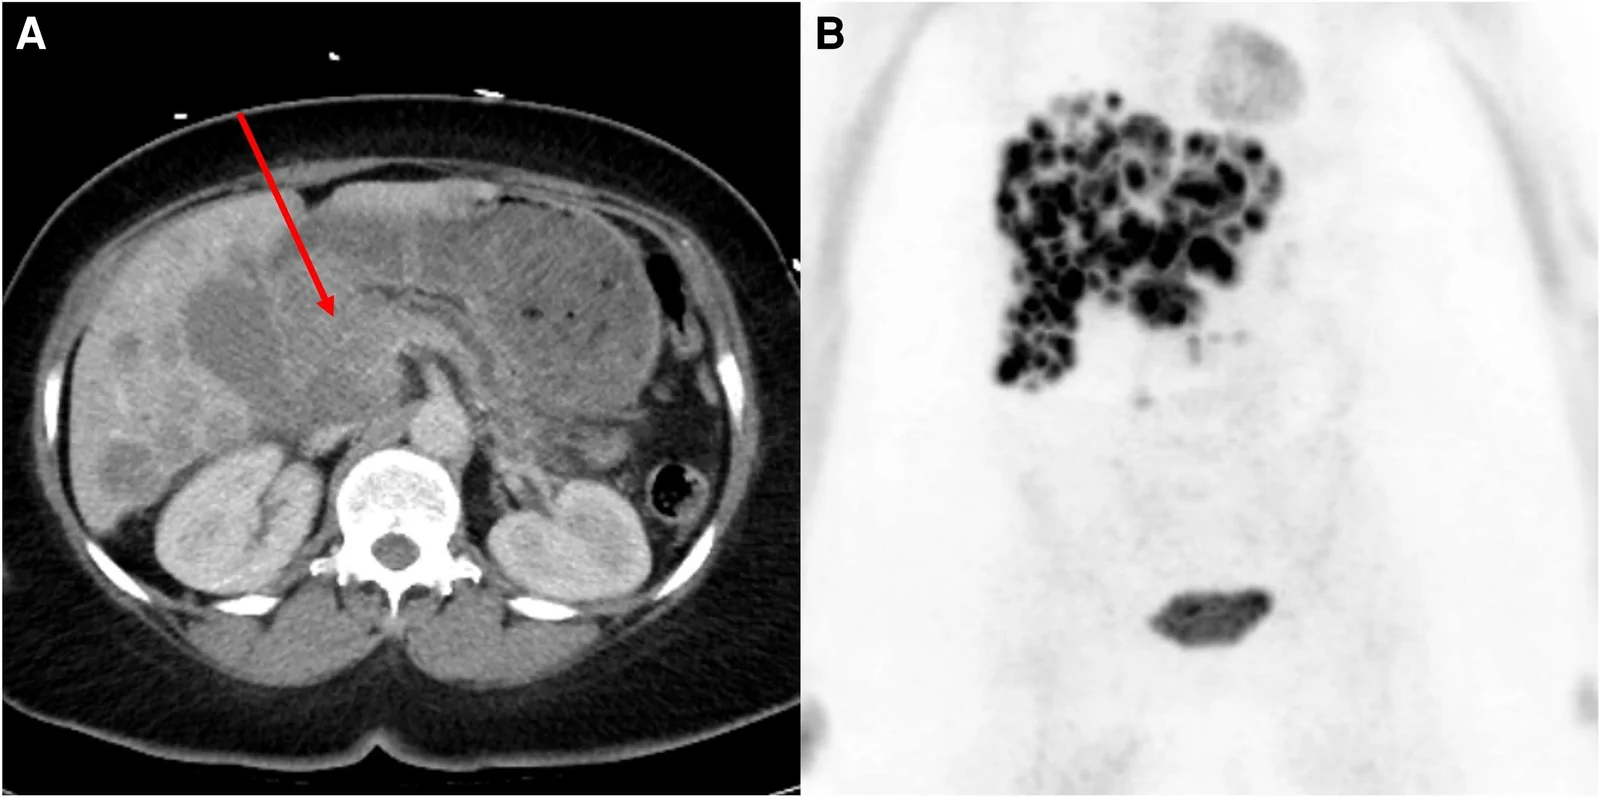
Imaging findings demonstrating metastatic pancreatic malignancy. (A) Axial computed tomography (CT) of the abdomen demonstrating a large hypodense mass in the pancreatic head (red arrow) with associated hepatic metastases (B) Fluorine-18 fluorodeoxyglucose positron emission tomography/computed tomography (PET/CT) showing numerous hypermetabolic lesions throughout the liver consistent with metastatic disease.
